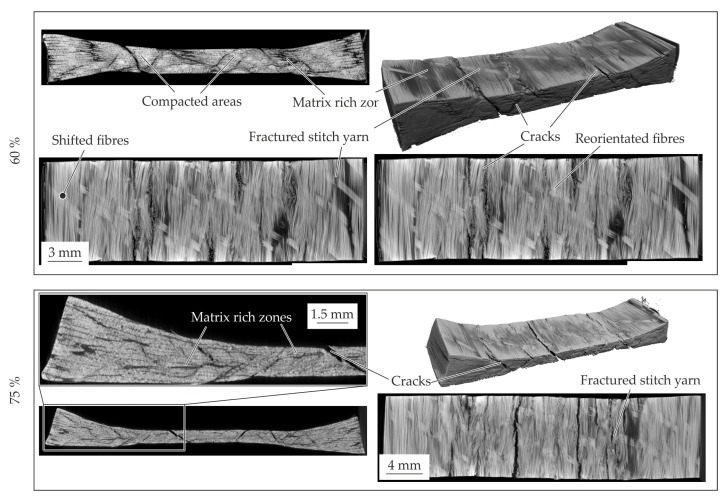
CT analysis of the 4 mm—configuration for the different compaction levels at 180 °C.
